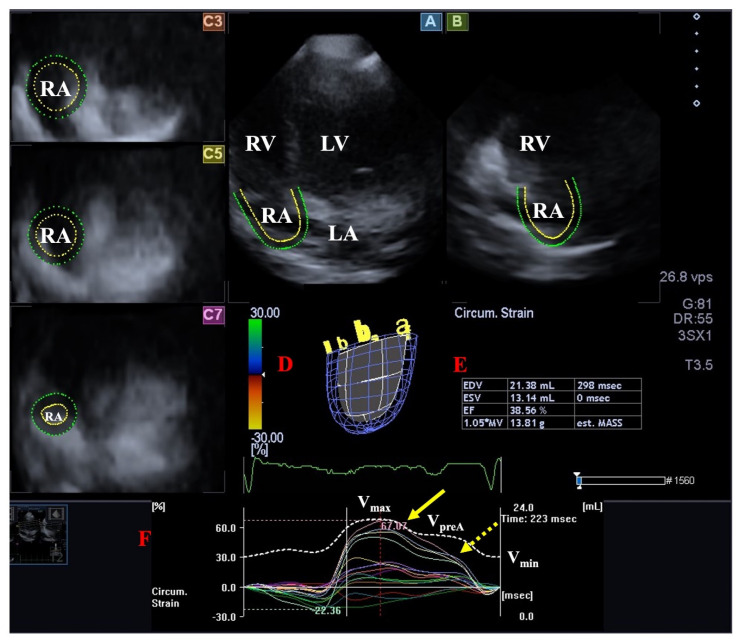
Evaluation of the right atrium (RA) by three-dimensional (3D) speckle-tracking echocardiography. Following acquisition of a 3D echocardiographic dataset and by using dedicated vendor-provided software, several views were produced automatically: Longitudinal apical four-chamber (A) and two-chamber (B) views and short-axis views at basal (C3), midatrial (C5), and superior (C7) RA levels. The 3D cast of the RA (D) is shown with calculated RA volumes (E) and time–RA global (white curve) and segmental (colored curves) longitudinal (F) RA strain curves together with a time–RA volume change curve (dashed white curve). The yellow arrow represents peak RA strains, whereas the yellow dashed arrow represents RA strains at atrial contraction. Abbreviations: LV = left ventricle, LA = left atrium, RV = right ventricle, RA = right atrium, EDV = end-diastolic volume, ESV = end-systolic volume, EF = ejection fraction, MASS = RA muscle mass, Vmax = minimum end-systolic RA volume, VpreA = early diastolic RA volume before atrial contraction, Vmin = end-diastolic minimum RA volume.
